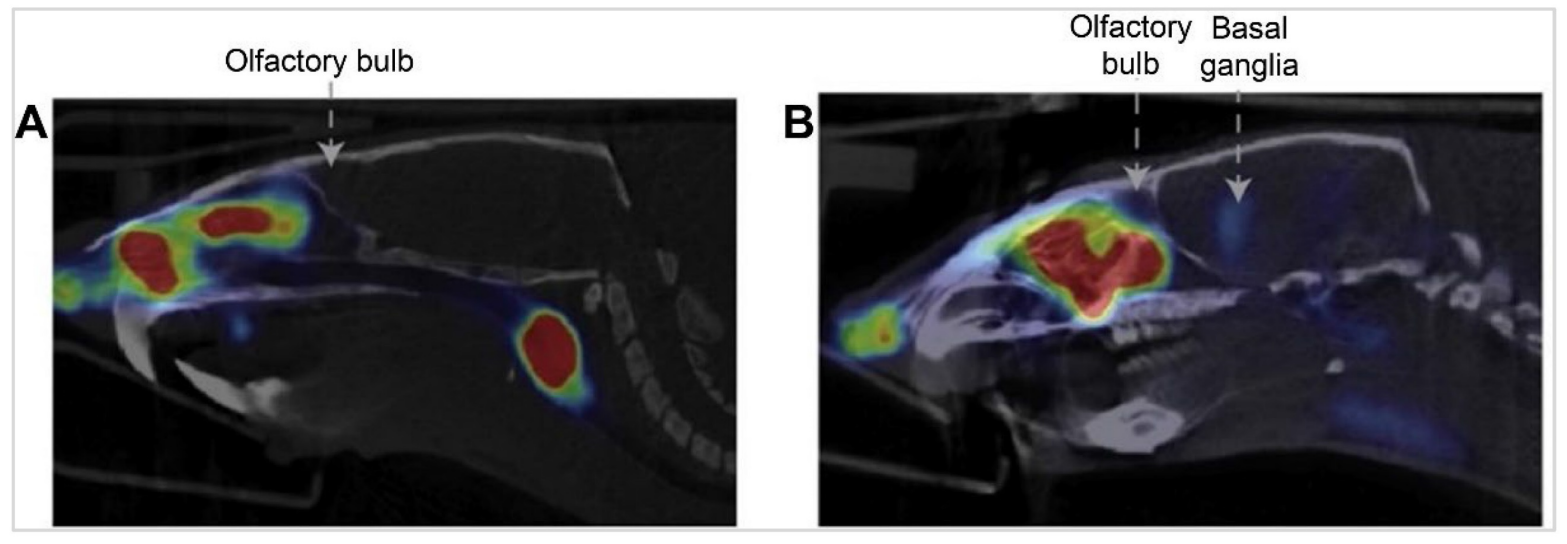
Presents lateral views of the brain following IN administration of (A) [^18^F]FDG and (B) [^18^F]fallypride. In both images, arrows point to the olfactory region. Additionally, the basal ganglia are indicated in the image (B) of [^18^F]fallypride. Adapted with permission from 86, copyright 2018 Elsevier.
